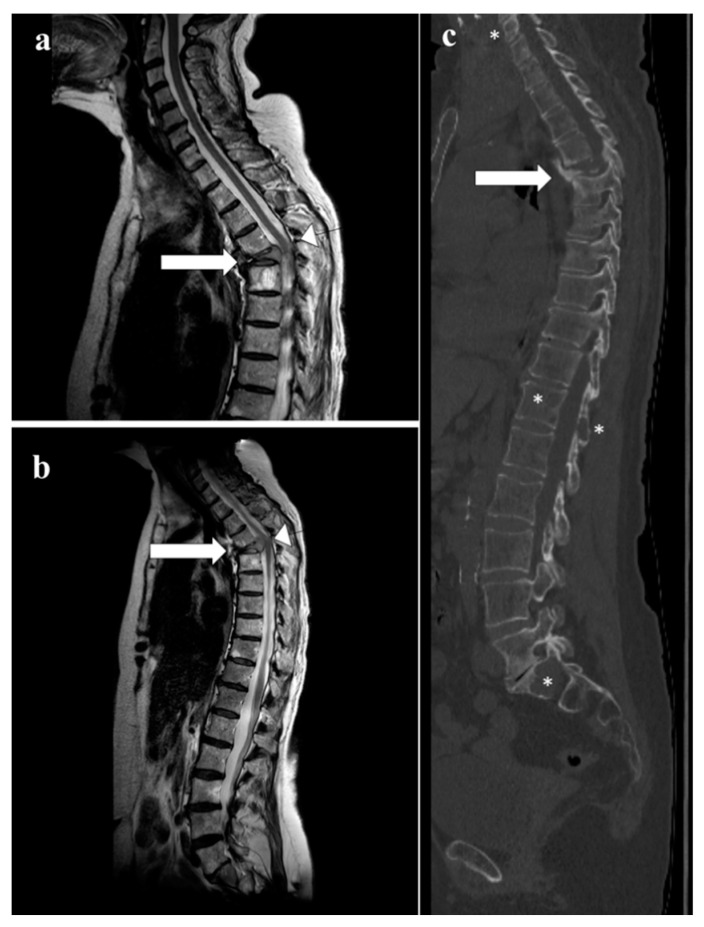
Multiple myeloma in a 74-year-old female, with pathological fracture of T5 vertebra and medullary compression as the first symptom. T2-weighted sagittal MRI of the cervicothoracic (a) and lumbar spine (b) showing an approximately 90% loss of vertebral height at T5 (white arrow) with canal compromise (small head-arrow). (c) Sagittal CT scan confirming the pathological fracture (white arrow) and multiple osteolytic lesions (white asterisks).
